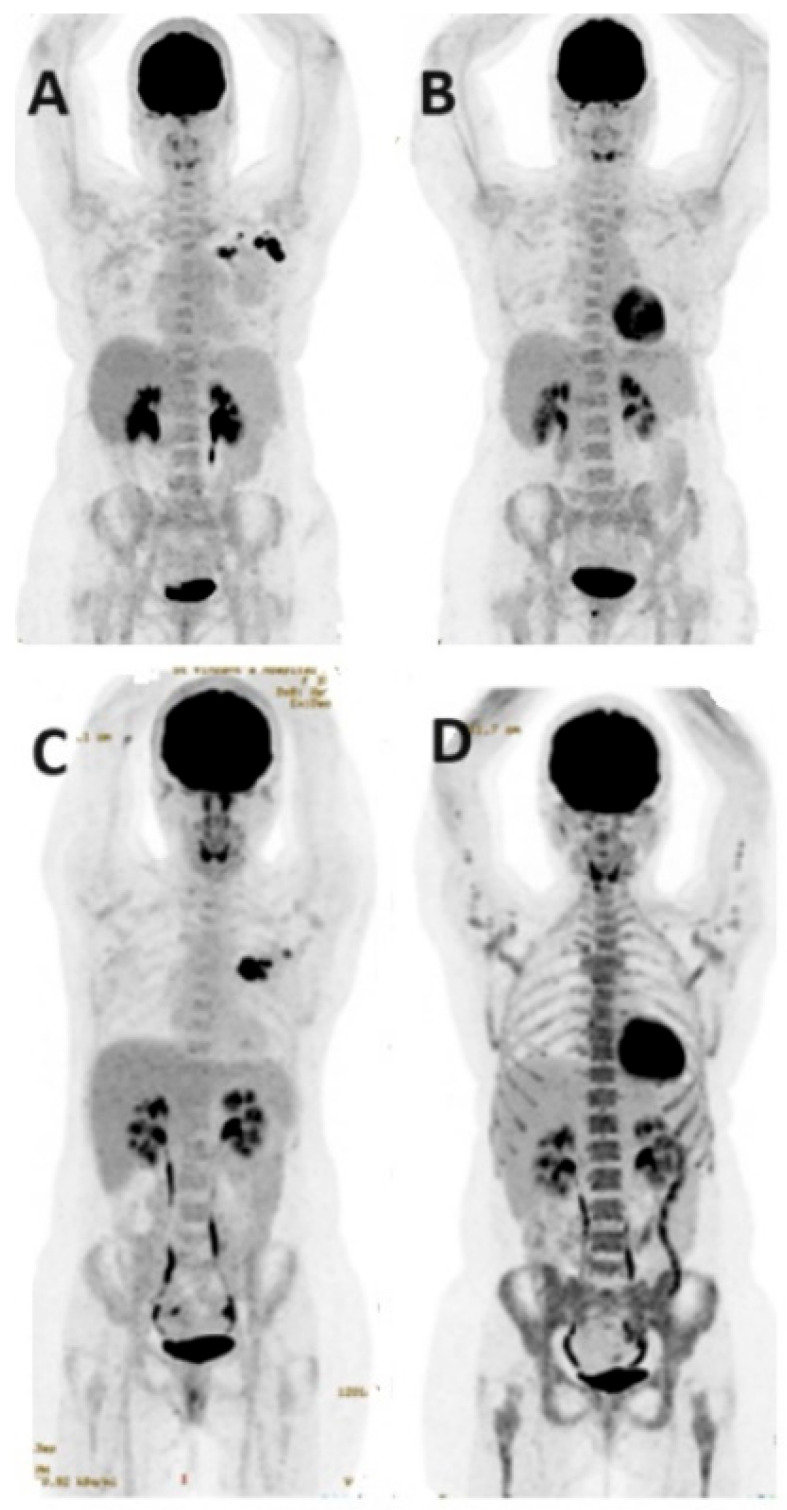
FDG PET/CT in response assessment. Baseline PET/CT MIP images (A,C) show FDG-avid left BC and ipsilateral axillary nodal metastases in two patients before neoadjuvant chemotherapy (NAC). Post-NAC PET/CT MIP images (B,D) demonstrate an excellent treatment response, with significantly reduced FDG uptake in the primary and axillary nodal metastases following neoadjuvant chemotherapy.
